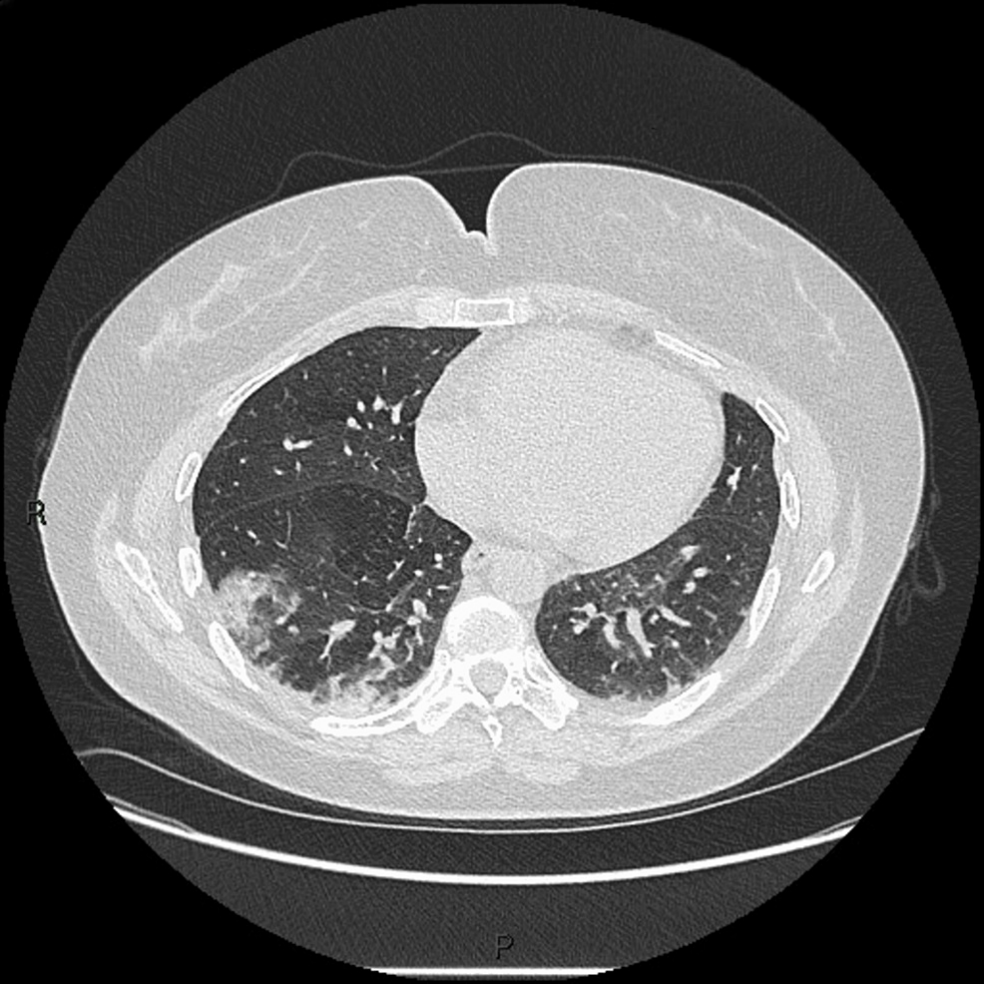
HRCT of patient 2 showing right middle lobe pneumonitis and ground-glass opacities HRCT: High-resolution computed tomography.
